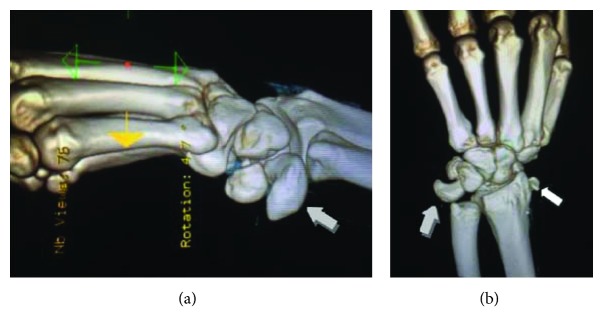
Preoperative 3D CT/scan of the left wrist showing trans-scaphoid volar dislocation of the lunate (grey arrow shows the dislocation of the lunate and the white arrow the proximal pole of the scaphoid).
